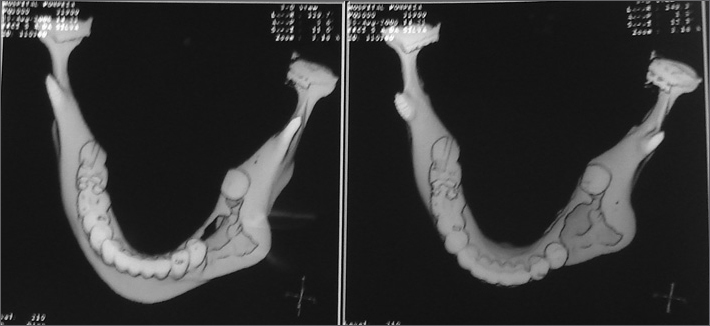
Mandible CT scan showing morphostructural alteration with bone remodeling and a large irregular lithic area in the body and alveolar processes of the left mandible.
